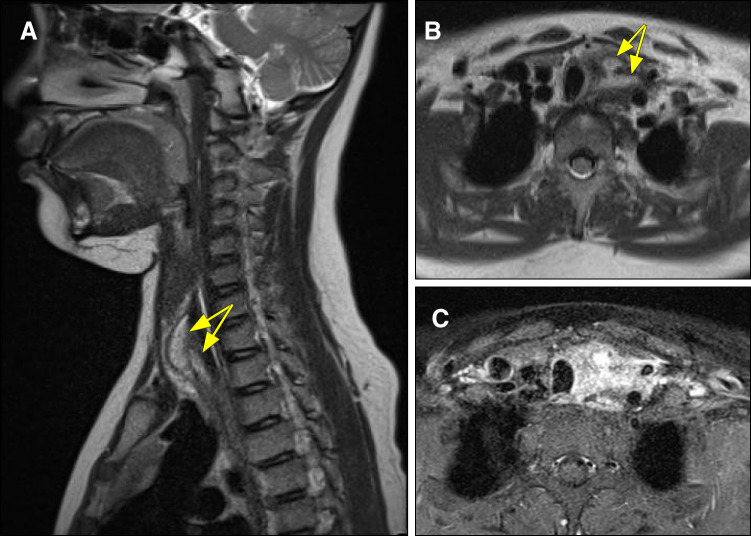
MRI findings. (A, B) T1-weighted enhanced MRI demonstrating a 22 × 20 × 46-mm cyst with constriction (arrows). (C) Contrast-enhanced MRI showing extensive peripheral enhancement around the cyst.
